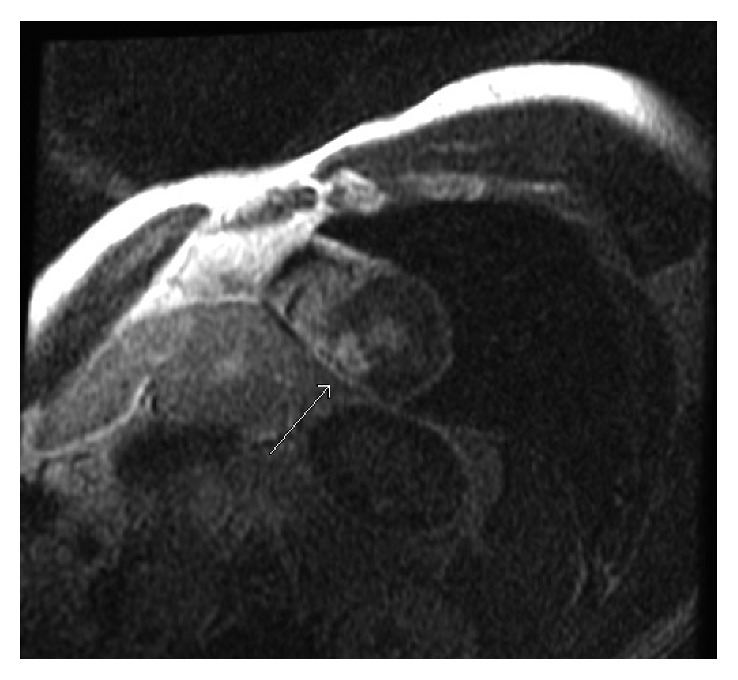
Delayed postcontrast cardiac MRI short axis view demonstrating midmyocardial enhancement of the inferior wall of the left ventricle adjacent to the apex. Subendocardial sparring is again seen.
